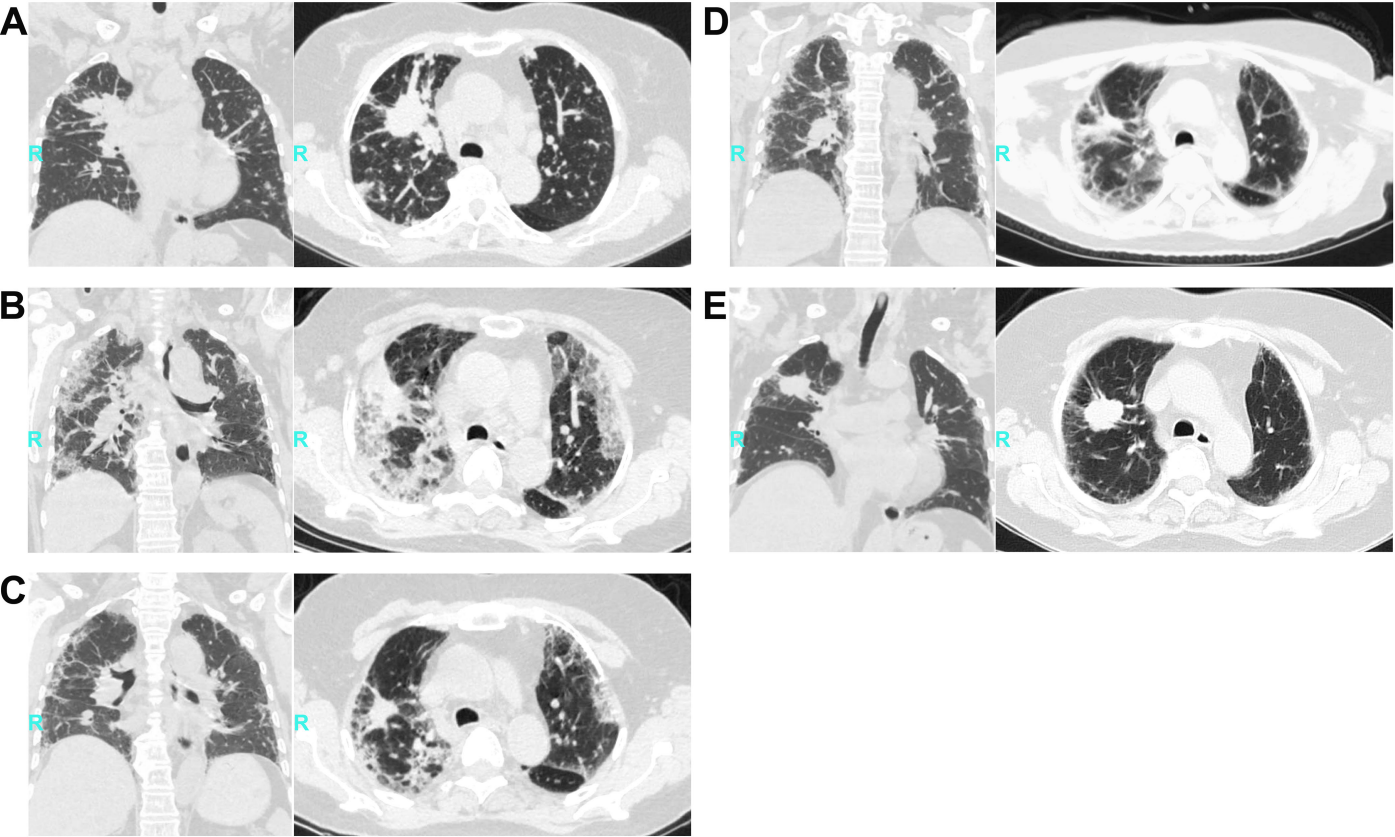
Sequential thoracic CT in EGFR-mutant lung adenocarcinoma with CMV pneumonitis. (All images: lung window [WW 1500, WL -600]). (A) Baseline (11 Sep 2023): Spiculated right hilar mass (47×37 mm) with interlobular septal thickening suggests lymphangitic carcinomatosis. (B) Acute pneumonitis (28 Dec 2023): Diffuse bilateral ground-glass opacities (GGOs) with septal thickening and reticulation. (C) Progressive damage (12 Jan 2024): Increased GGO density with peribronchovascular crazy-paving pattern. (D) Fibrosing phase (18 Feb 2024): Subpleural and peribronchovascular consolidations with reticulation. BAL confirmed CMV viremia (20,000 copies/mL). (E) Post-inflammatory fibrosis (1 May 2024): Irreversible architectural distortion with traction bronchiectasis. BAL, bronchoalveolar lavage; CMV, cytomegalovirus; DAD, diffuse alveolar damage; EGFR, epidermal growth factor receptor; GGO, ground-glass opacity; TKI, tyrosine kinase inhibitor; WL, window level; WW, window width.
